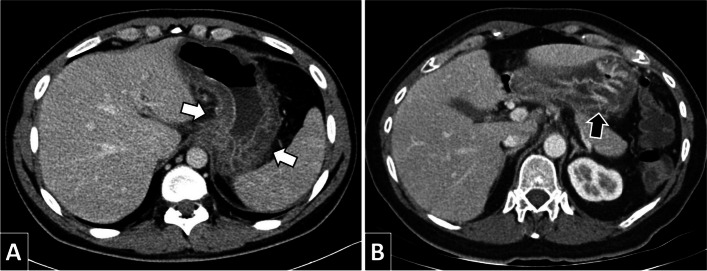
Patients of 31 (A) and 50 years (B) of age with gastric anisakiasis by CT. The diffuse thickening of the gastric wall with marked submucosal oedema (A, white arrows), as well as the oedema of the folds (B, black arrow) can be observed
